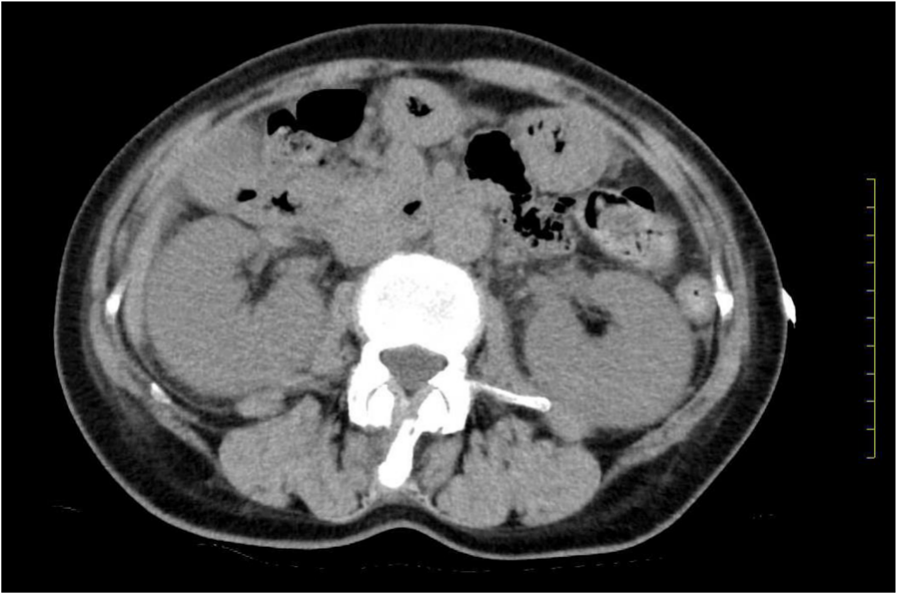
Abdominal computed tomography (CT) showing slight enlargement of both kidneys (right, 12 × 7 cm; left, 11 × 6 cm)
